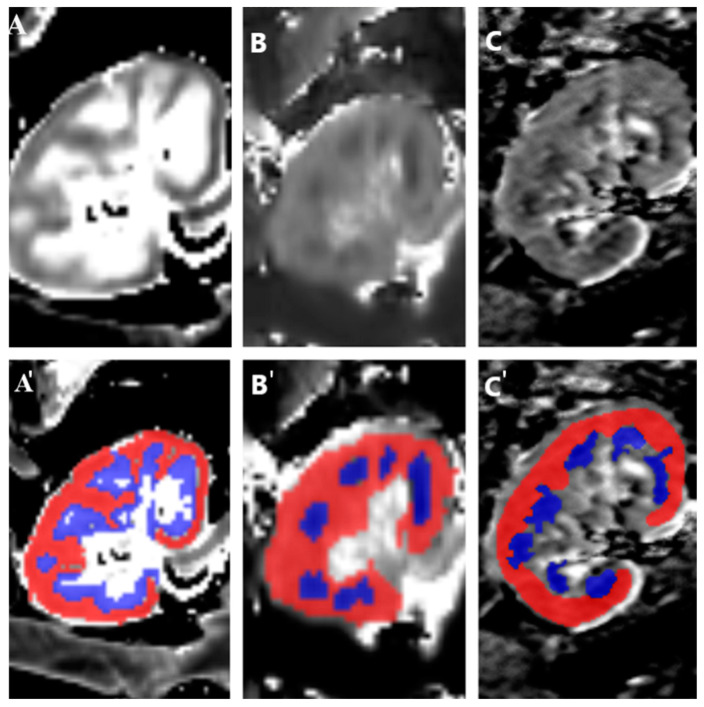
The post-processing images of transplanted kidneys: T1 map (A), T2 map (B), and ADC map (C). Segmentation of renal MRI data. The cortex and medulla were identified map, and ROIs were semi-automatically delineated in the cortex (red) and medulla (blue) on the T1 map (A’), T2 map (B’), and ADC map (C’).
